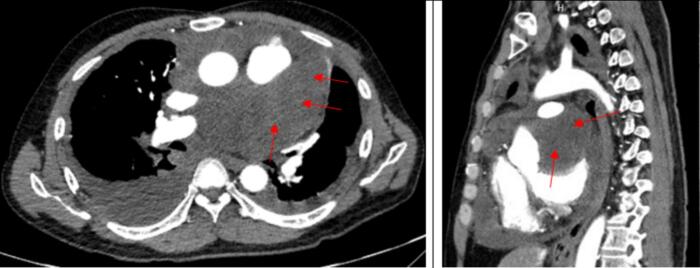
Chest CT (September 2023): bilateral pleural effusion, large tumor in the base area around large blood vessels, pericardial effusion.
